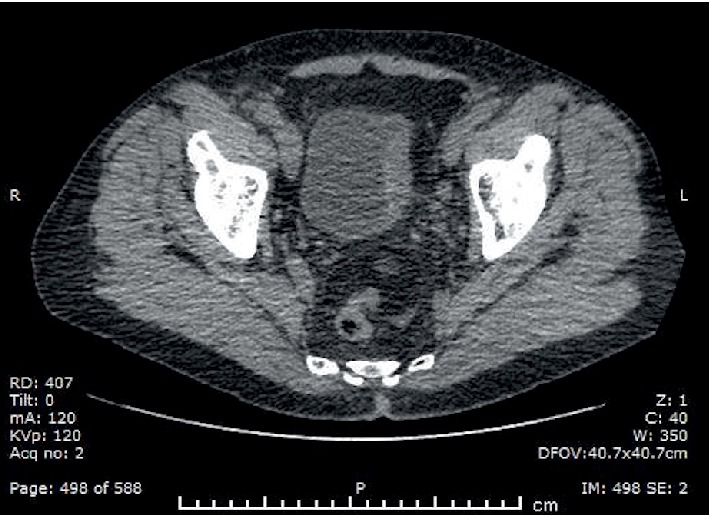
Computed tomography image of a bladder tumour on the left bladder wall.
